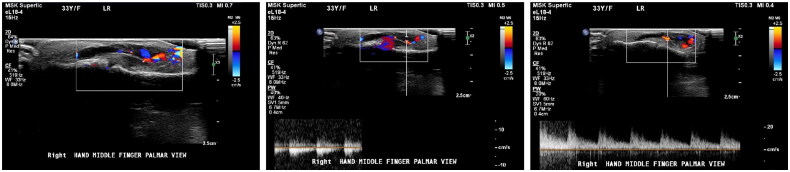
Ultrasound of the right Middle finger.
